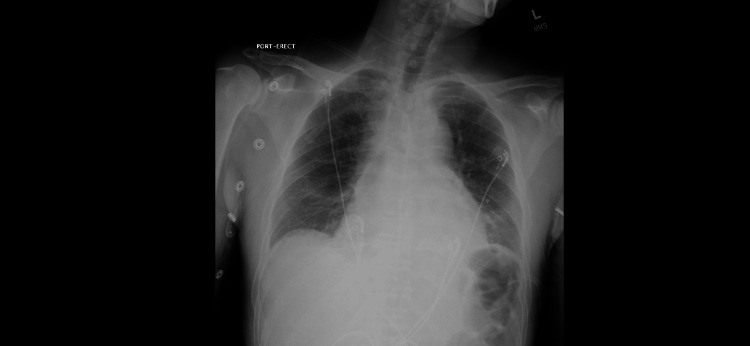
The chest X-ray showed mild pulmonary congestion and small pleural effusions.
